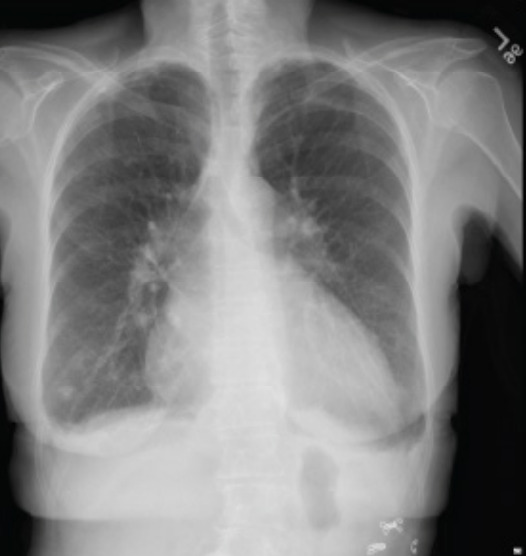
Chest radiograph of a 70-year-old woman with chest tightness and bradycardia.
